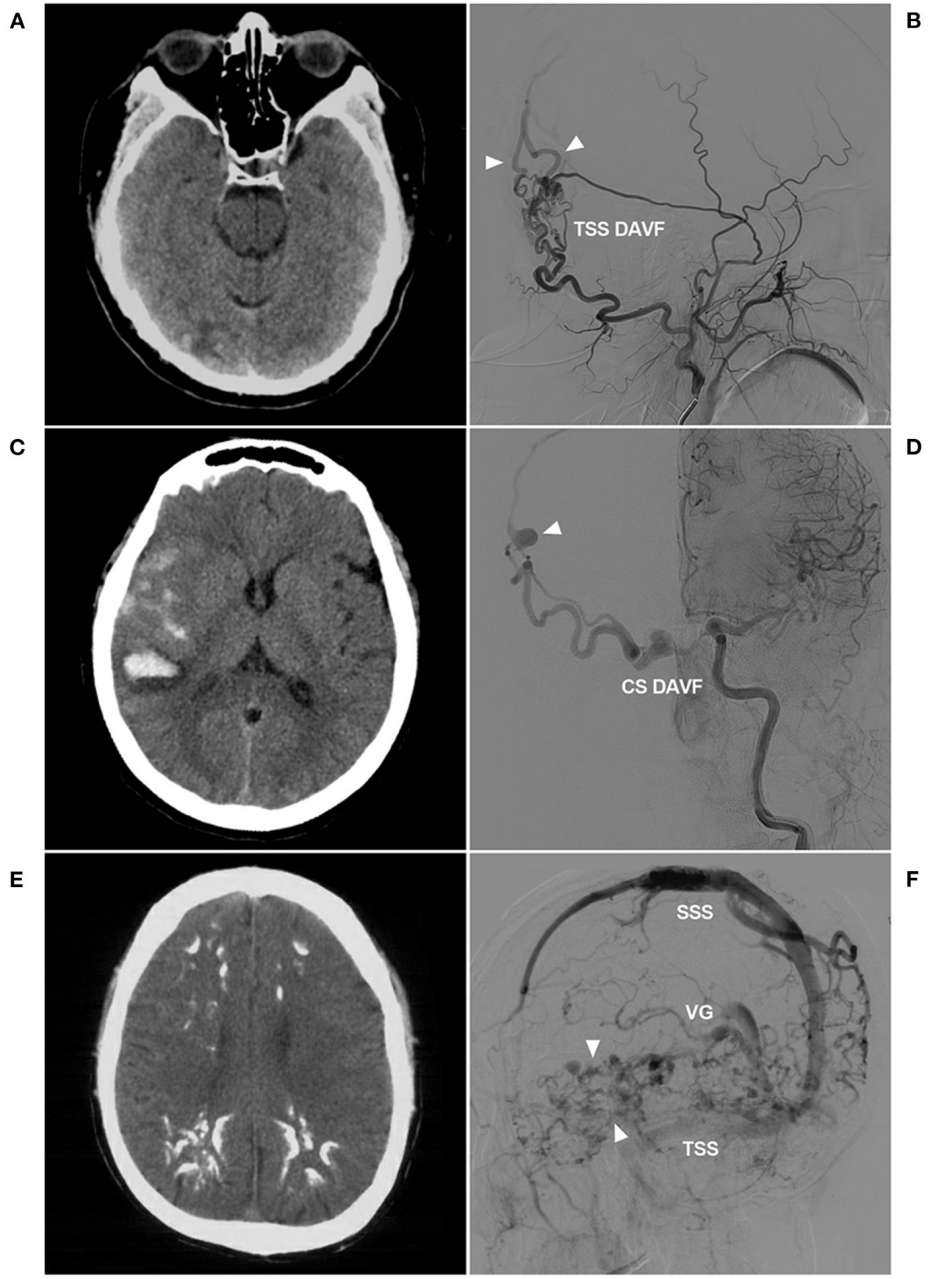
Venous drainage patterns of DAVFs. (A,B) Unruptured DAVF with images from the same patient. CT (A) showed a mix of high and low densities in the right occipital lobe. DSA (B) showed a TSS DAVF draining to multiple cortical veins (triangles), and the veins throughout the entire brain were normal and not dilated. (C,D) Hemorrhagic DAVF with images from the same patient. CT (C) showed subarachnoid hemorrhage and hematoma in the Sylvian fissure region. DSA (D) showed a CS DAVF with cortical vein drainage and a ruptured venous aneurysm (triangle). (E,F) Extensive pseudophlebitic DAVF with images from the same patient. CT (E) showed multiple calcifications in the white matter around the ventricle. DSA (F) showed an extensive pseudophlebitic pattern involving superficial and deep veins (triangles). CS, cavernous sinus; CT, computed tomography; DAVF, dural arteriovenous fistula; DSA, digital subtraction angiography; SSS, superior sagittal sinus; TSS, transverse-sigmoid sinus; VG, vein of Galen.
